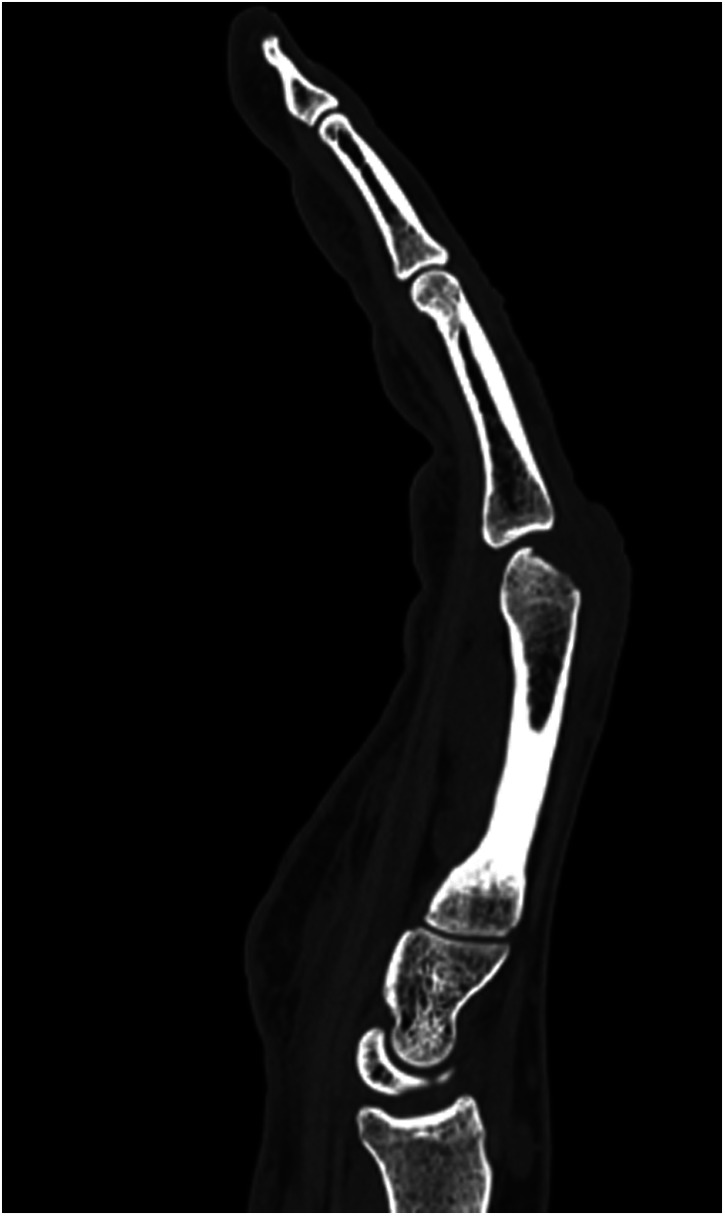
Case 3 preoperative CT imaging of dorsal defect of the third metacarpal head.
